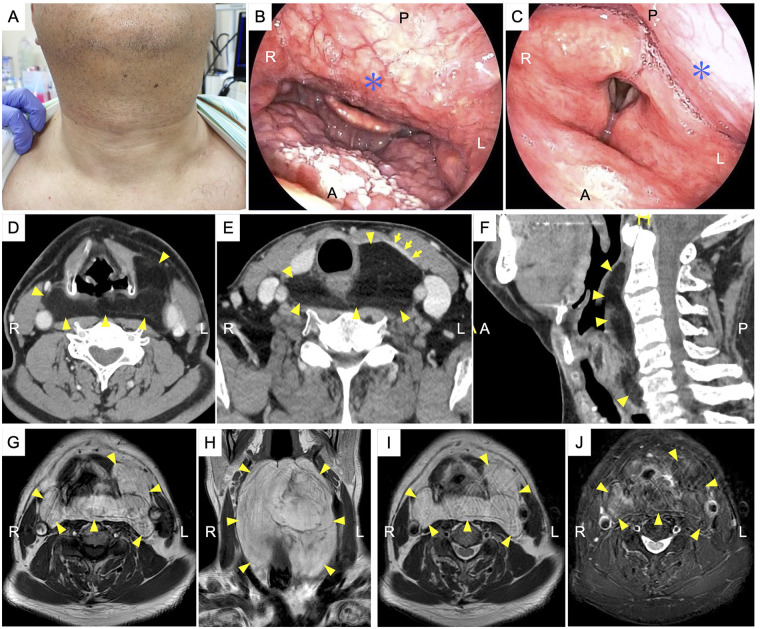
Physical examination, endoscopic evaluation, and imaging findings on computed tomography (CT) and magnetic resonance imaging (MRI). (A) Physical examination demonstrates diffuse subcutaneous swelling of the neck. (B,C) Flexible endoscopic findings revealing submucosal bulging (*) of the posterior pharyngeal wall from the oropharynx (B) to the hypopharynx (C). (D–F) CT images displaying a well-circumscribed, non-enhanced, fat-density mass (arrowheads) in the retropharyngeal space, extending vertically from the level of the uvula to the lower pole of the thyroid gland (F), displacing the pharyngolarynx, trachea, esophagus, and thyroid gland, particularly the left lobe ((E), arrows) anteriorly, and the common carotid arteries bilaterally (D,E). (G–J) MRI displaying a lesion (arrowheads) with thin septations, exhibiting hyperintense signal on both T1-weighted (G,H) and T2-weighted (I) images, with obvious signal suppression on fat-saturated sequences (J), consistent with the diagnosis of lipoma. (R, right; L, left; A, anterior; P, posterior).
